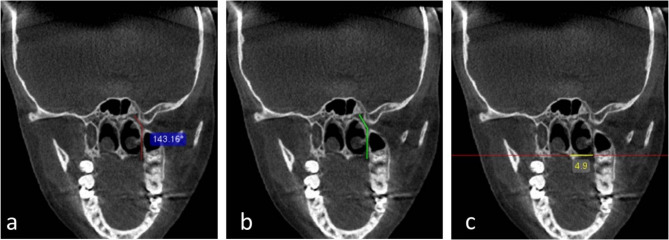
a Coronal CBCT reformatted image showing the angle formed by the GPC pathway from PPF axis to GPF (red curved line). b coronal CBCT reformatted image showing GPC pathway type (B) (green curved line). c Coronal CBCT reformatted image showing GPF-MMS distance (yellow line)For GPC pathways the following types were developed:AGPC travels directly in an inferior direction through its whole pathway.BGPC travels in an inferior-lateral direction, then directly inferior (Fig. 2b).CGPC travels in an inferior-lateral direction, then changes its path into an inferior-medial direction.DOther
